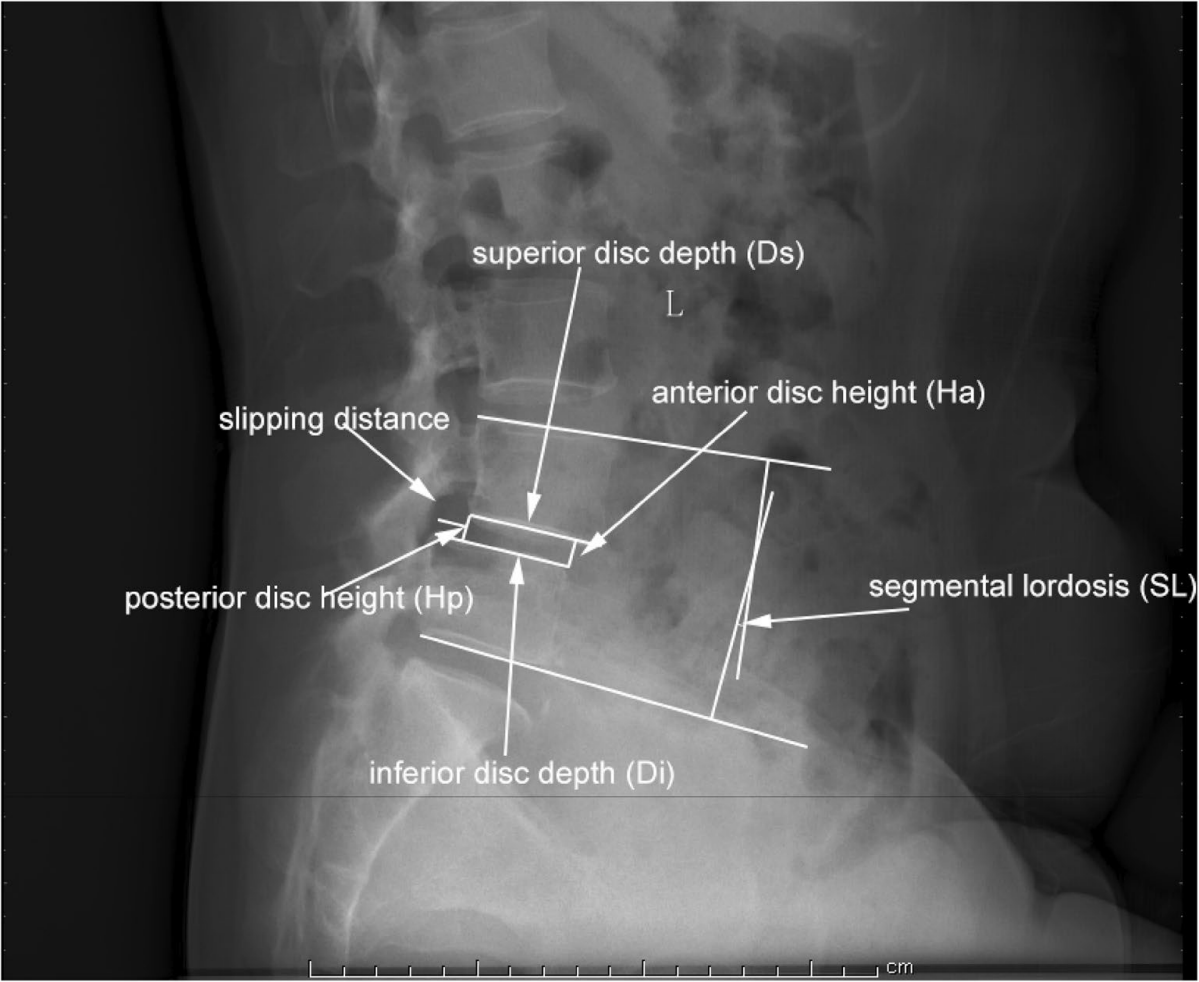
Parameters measured on X-ray radiographs. Anterior disc height index (DHIA) = [Ha/(Ds + Di)] *100%. Posterior disc height index (DHIP) = [Hp/(Ds + Di)] *100%.
